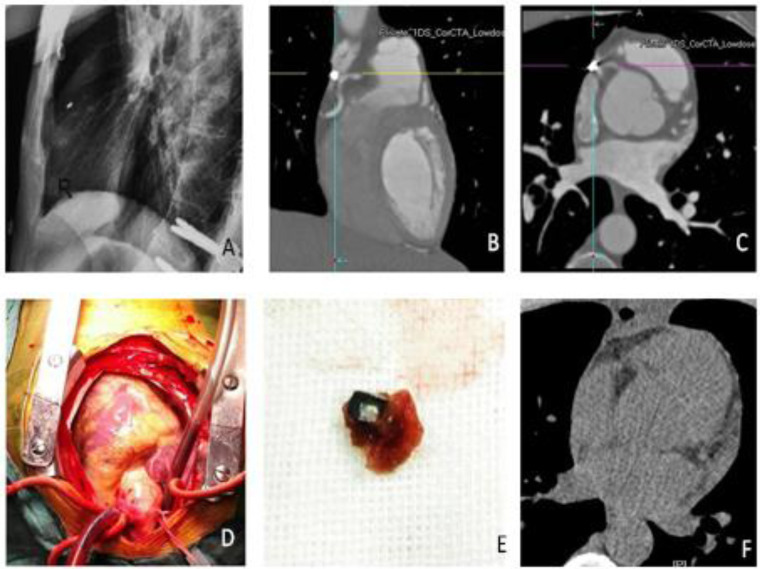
Radiation and dual-source CTA showed that the rongeur tip was in the auricula dextra (A–C). The tip was found in the auricula dextra close to the right atrioventricular groove (D). Image of the extracted rongeur tip (E). CT was used to confirm (F).
